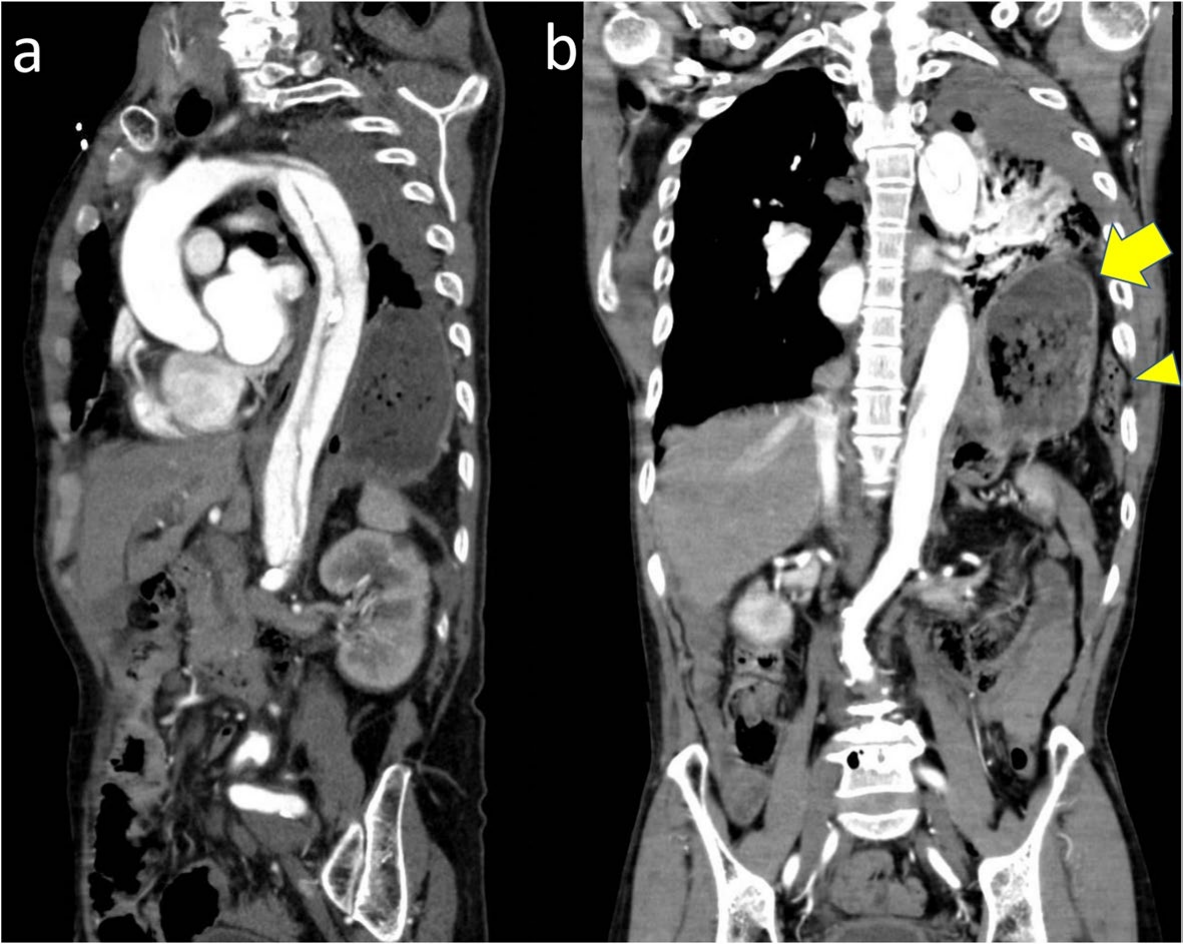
Contrast-enhanced computed tomography images at hospital arrival. Computed tomography showing aortic dissection from the level of the left subclavian artery to the celiac artery (a). In the coronal section, diaphragmatic rupture and herniation of the stomach (arrow), descending colon (arrow head) into the left thoracic cavity, and hemothorax are observed (b)
